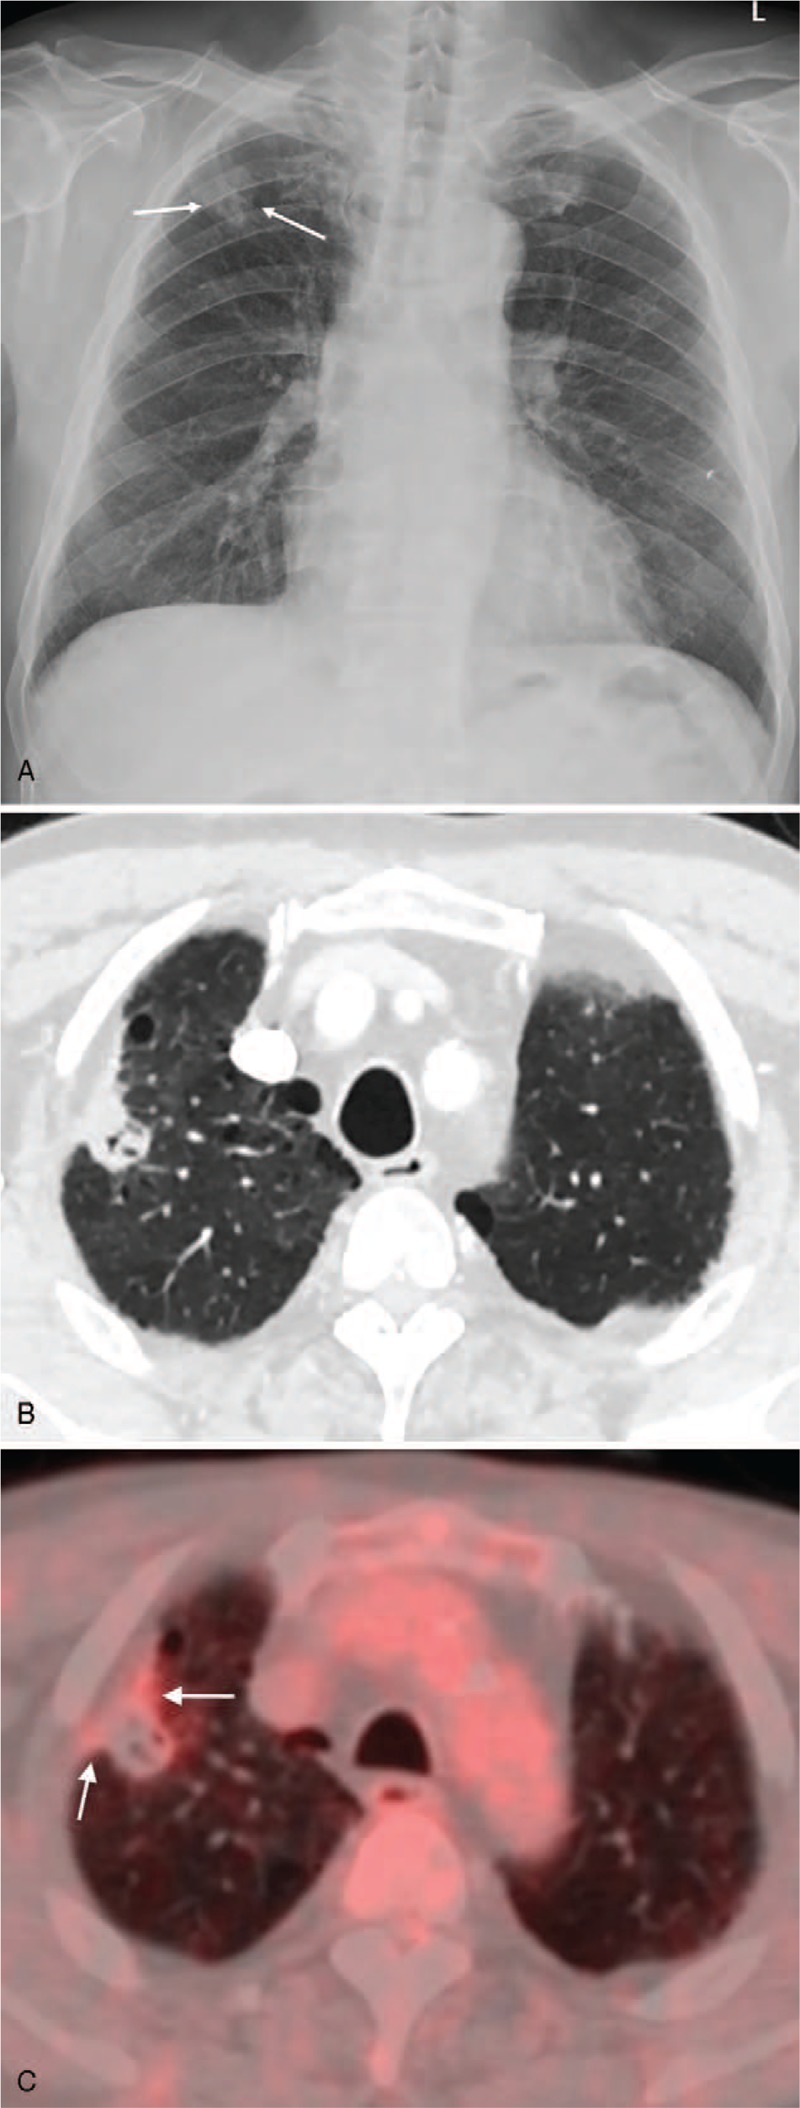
A 73-year-old male patient presented with incidental lung nodule. A. Chest X-ray showing a nodule overlapping with the 2nd rib (arrows) in the right upper lobe. B. Chest CT scan showing a cavitary nodule with eccentric wall thickening and adjacent pleural retraction. C. F-18-FDG-PET/CT scan revealing hypermetabolic activity (SUVmax = 2.8, arrows) at the pleural side of the lesion.
